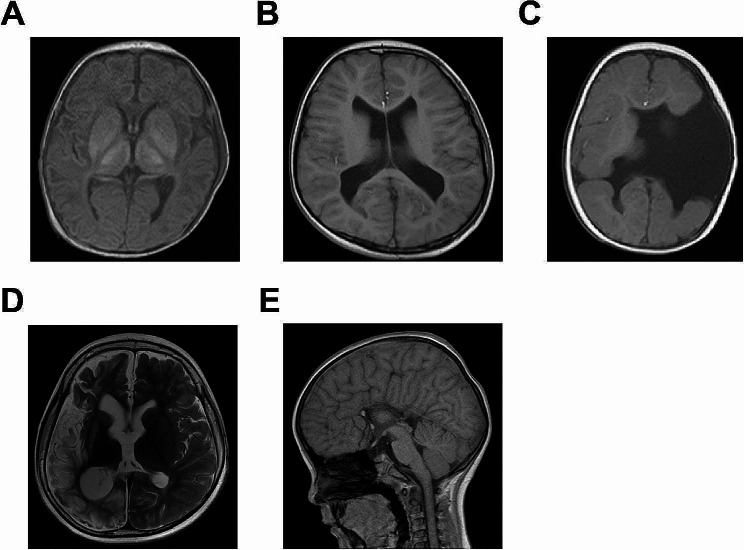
Representative MRI images of children with cerebral palsy. (A) Image of a child with cerebral palsy with cerebral ischemia. (B) Image of a child with cerebral palsy with encephalomalacia. (C) Image of a child with cerebral palsy with schizencephaly. (D) Image of a child with cerebral palsy with cerebral atrophy. (E) Image of a child with cerebral palsy with agenesis of the corpus callosum
